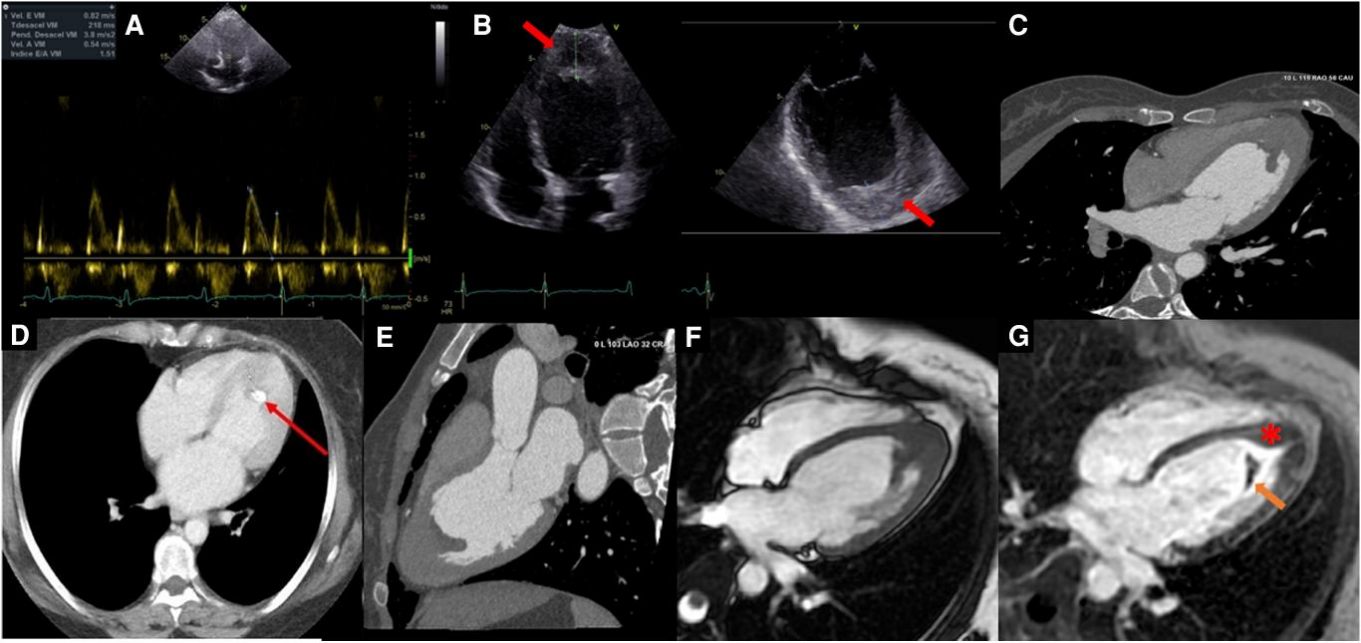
Case 3 images. (*A*) Pulsed Doppler demonstrated a restrictive filling pattern of the left ventricle and tissue Doppler with an e-wave at 4 cm/s (not shown). (*B*) Transthoracic echocardiogram. Four-chamber apical view echocardiogram with 27 mm apical thickening and endocardial calcification (red arrow). Transoesophageal echocardiography showed apical thickening and calcified areas (red arrow). (*C–E*) Cardiac computed tomography in four-chamber and three-chamber views showing apical obliteration, low attenuation LV filling defect consistent with LV thrombus, and calcification (arrow). A small left ventricular cavity is also seen. (*F*, *G*) Cardiac magnetic resonance in steady-state free precession and late gadolinium enhancement, respectively, showing apical diastolic obliteration (asterisk) and the ‘double V sign’ with endocardial enhancement and superimposed apical thrombus (orange arrow); in addition, diffuse intramyocardial enhancement is shown in the hypertrophied apical region.
